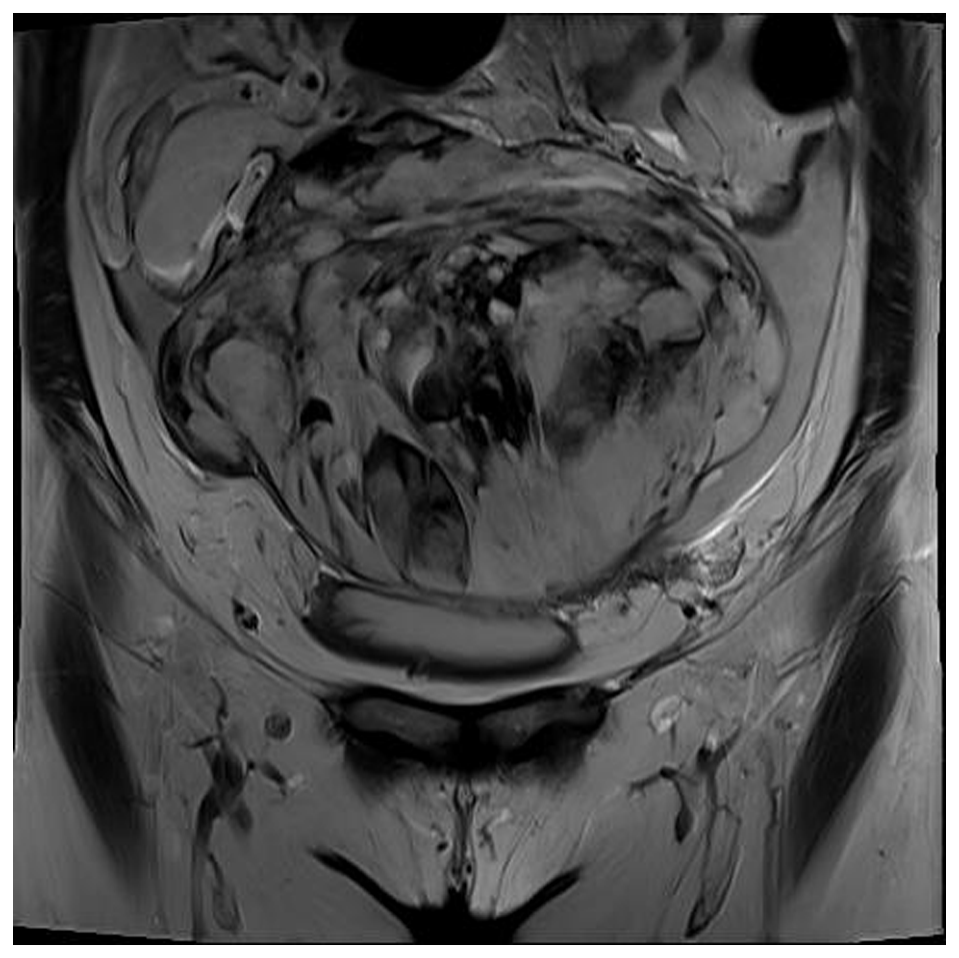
Magnetic resonance imaging (coronal view, T2 W1). This scan shows an approximately 15- × 9- × 17-cm mass with mixed high and low signal intensity and irregular margins in the lower abdomen on coronal view.
